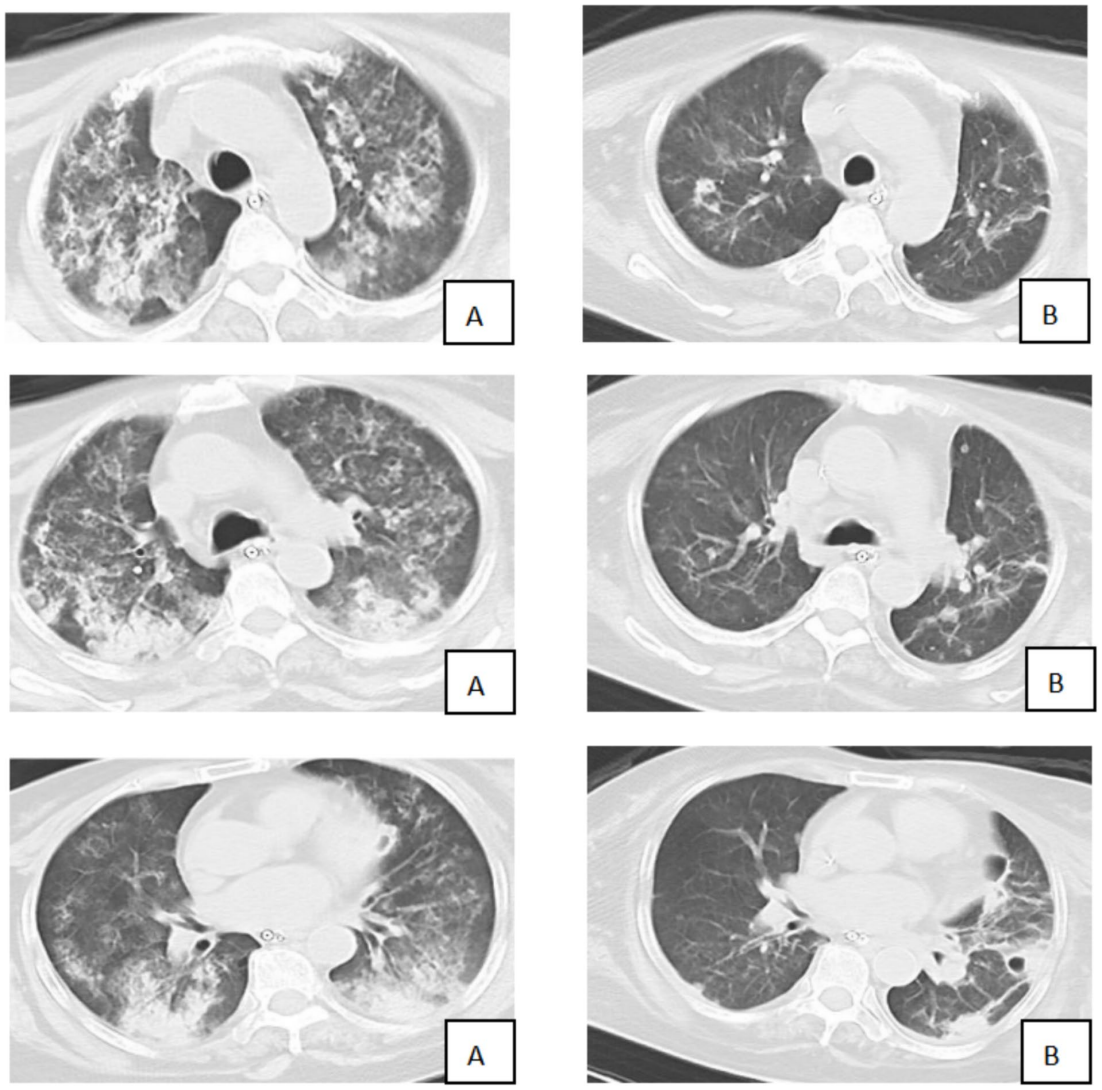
Comparison of chest CT before and after treatment (A: 1st August; B: 8th August).
